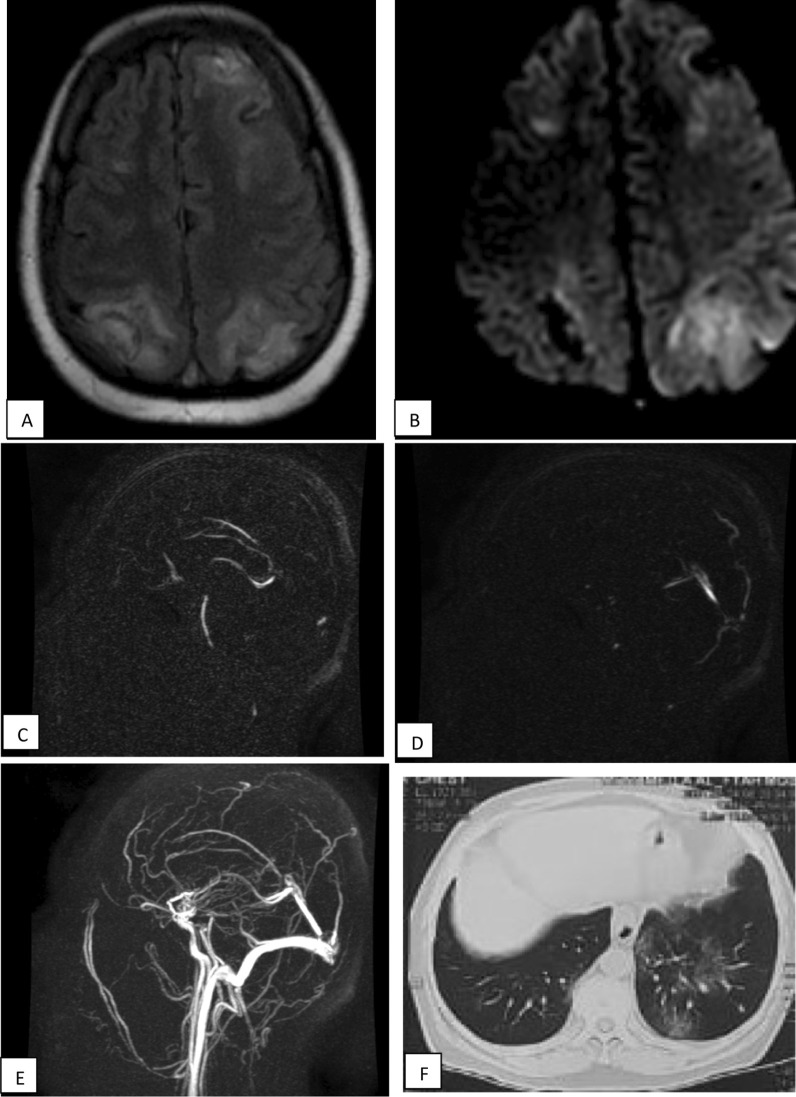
A 36-year-old male patient, presented with post-COVID headache, seizers and disturbed consciousness. a MRI axial T2WI revealed cortical and subcortical regions of high T2-signal intensities represents non haemorrhagic venous infarctions. b Corresponding regions of restricted diffusion in DWI with b-value1000. c, d and e MRV show near complete obstruction of the superior sagittal sinus. Non haemorrhagic venous infarction is confirmed. f CT chest showed peripheral distributed ground glass opacities typical for COVID-19 (CORAD-V)
